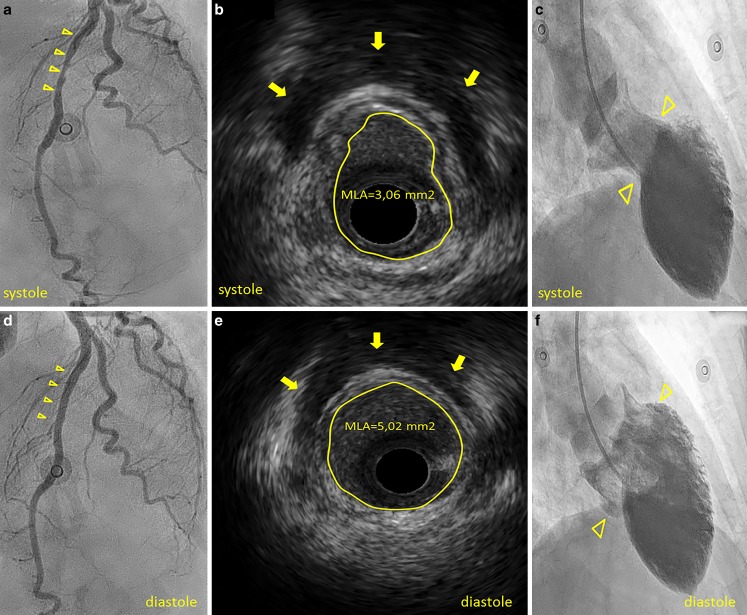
Wrap-around LAD with myocardial bridging in the mid segment (a in systole, d in diastole, *arrowheads*). Intravascular ultrasound demonstrating systolic compression of the mid-LAD with a mean lumen area oscillating from 3.06 mm^2^ in systole (b) to 5.02 mm^2^ in diastole (e) and an echolucent region between the bridged coronary segment and epicardial tissue persisting throughout the cardiac cycle, ‘half-moon sign’ (b, e, *arrows*). Left ventricular angiography revealing mid-apical ballooning with hypercontractility of the basal segments (c, f, *arrowheads*, in systole and diastole respectively)
